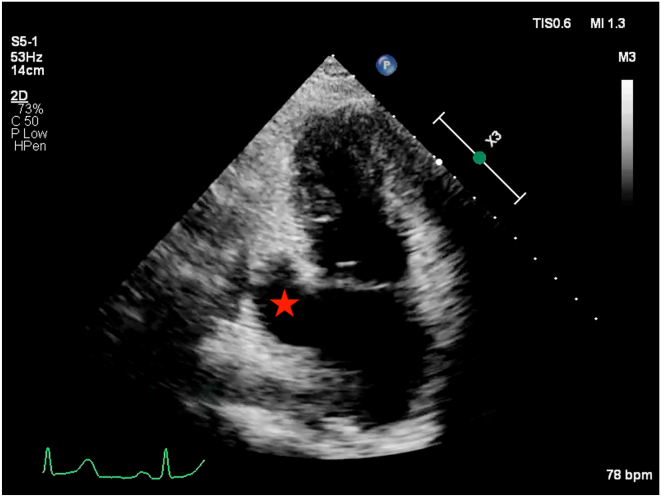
Two-dimensional TTE, apical 2-chamber systolic view, demonstrates the dilated CS with a deficient LA floor *(star)*.
